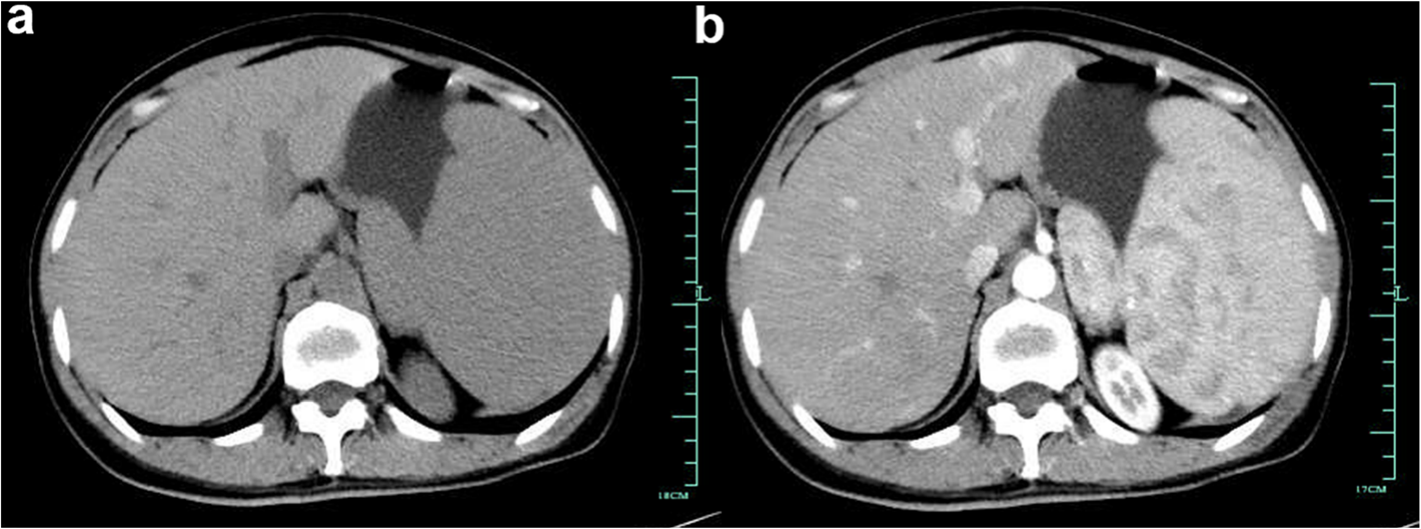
Abdominal enhanced computed tomography (CT) images. The images showed the presence of splenomegaly (a), without significant enhancement (b)
